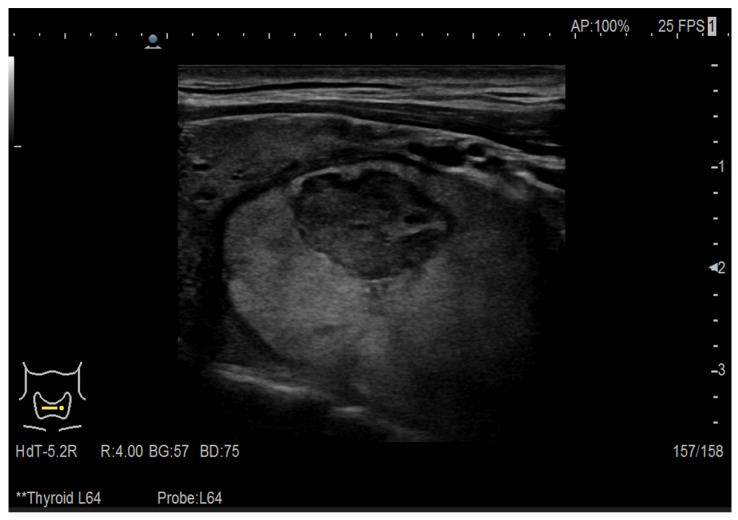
Ultrasound image of a thyroid with nodule.
